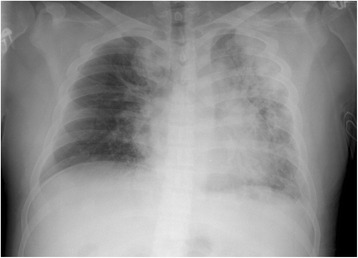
Chest x-ray. Chest x-ray showing massive diffuse parenchymal infiltration, predominantly of the left lung
